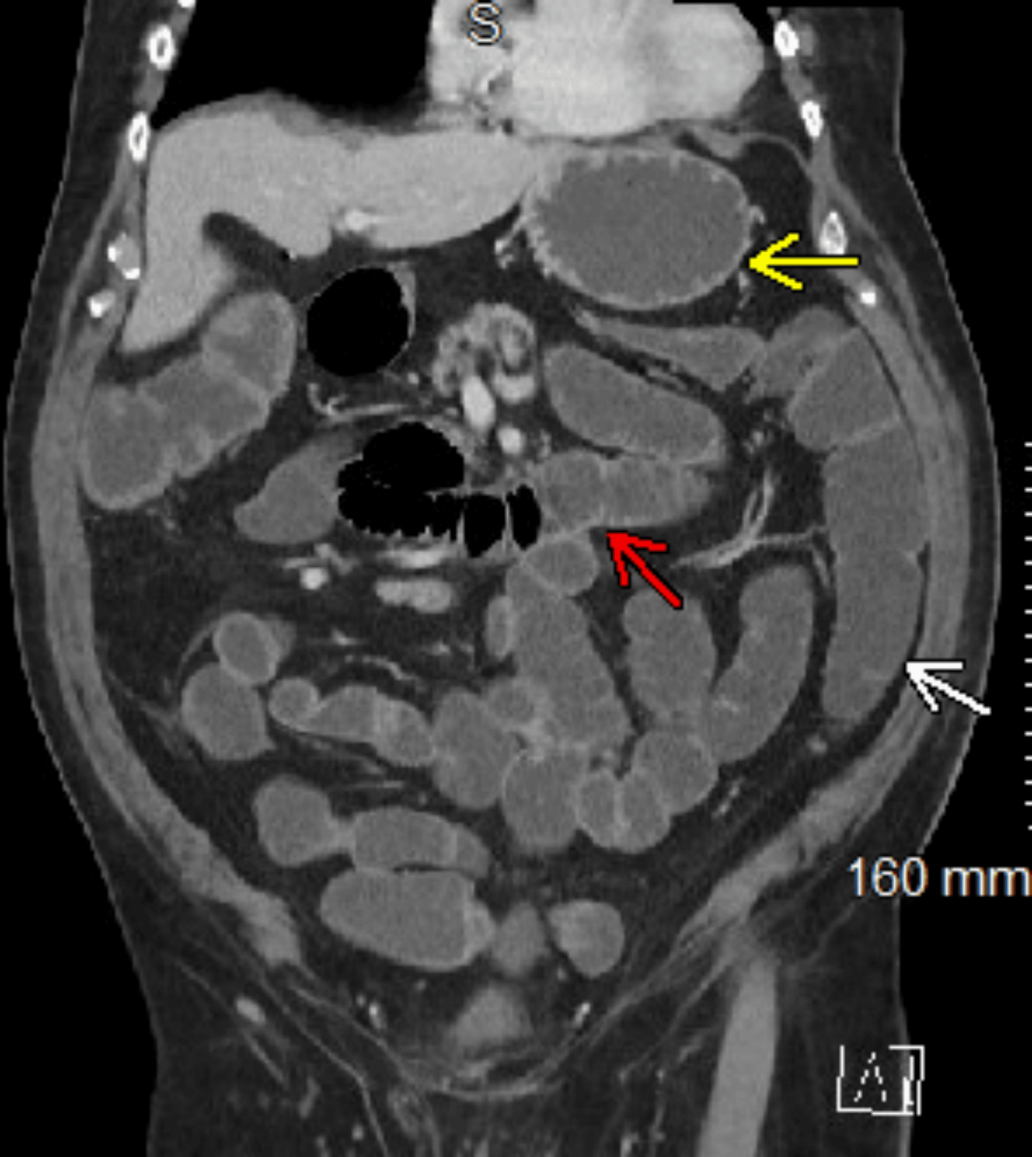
CT abdomen and pelvis showing fluid and gas distension of the stomach (yellow arrow), small intestine (red arrow), and colon (white arrow)
